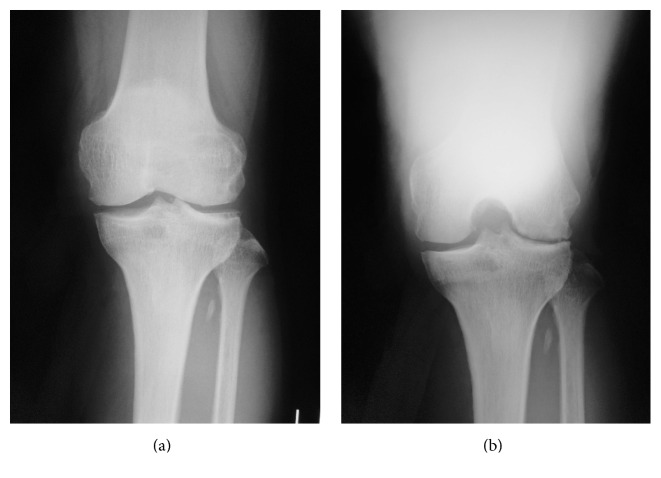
Radiographs of Case 1. AP view (a) shows grade 3 osteoarthritis according to Kellgren and Lawrence scale and Rosenberg view (b) shows lateral-dominant osteoarthritis.
